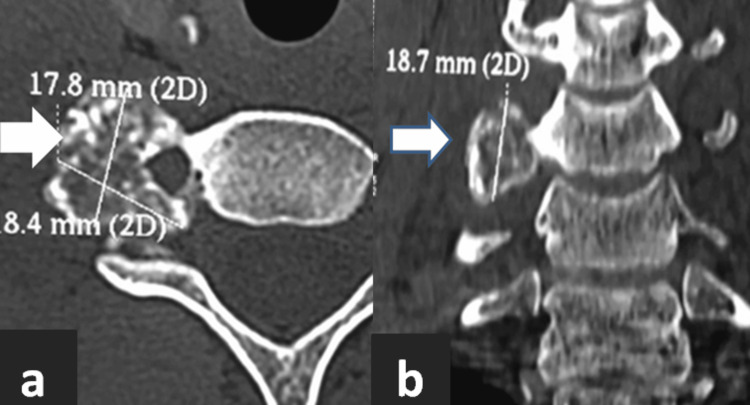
Preoperative CT showing a well-defined, lobulated, mixed lytic-sclerotic tumor mass measuring approximately 1.8 cm × 1.7 cm × 1.8 cm, originating from the right transverse process of the C6 vertebra. (a) Axial section CT image; (b) coronal section CT image CT: computed tomography; C6: sixth cervical vertebra
